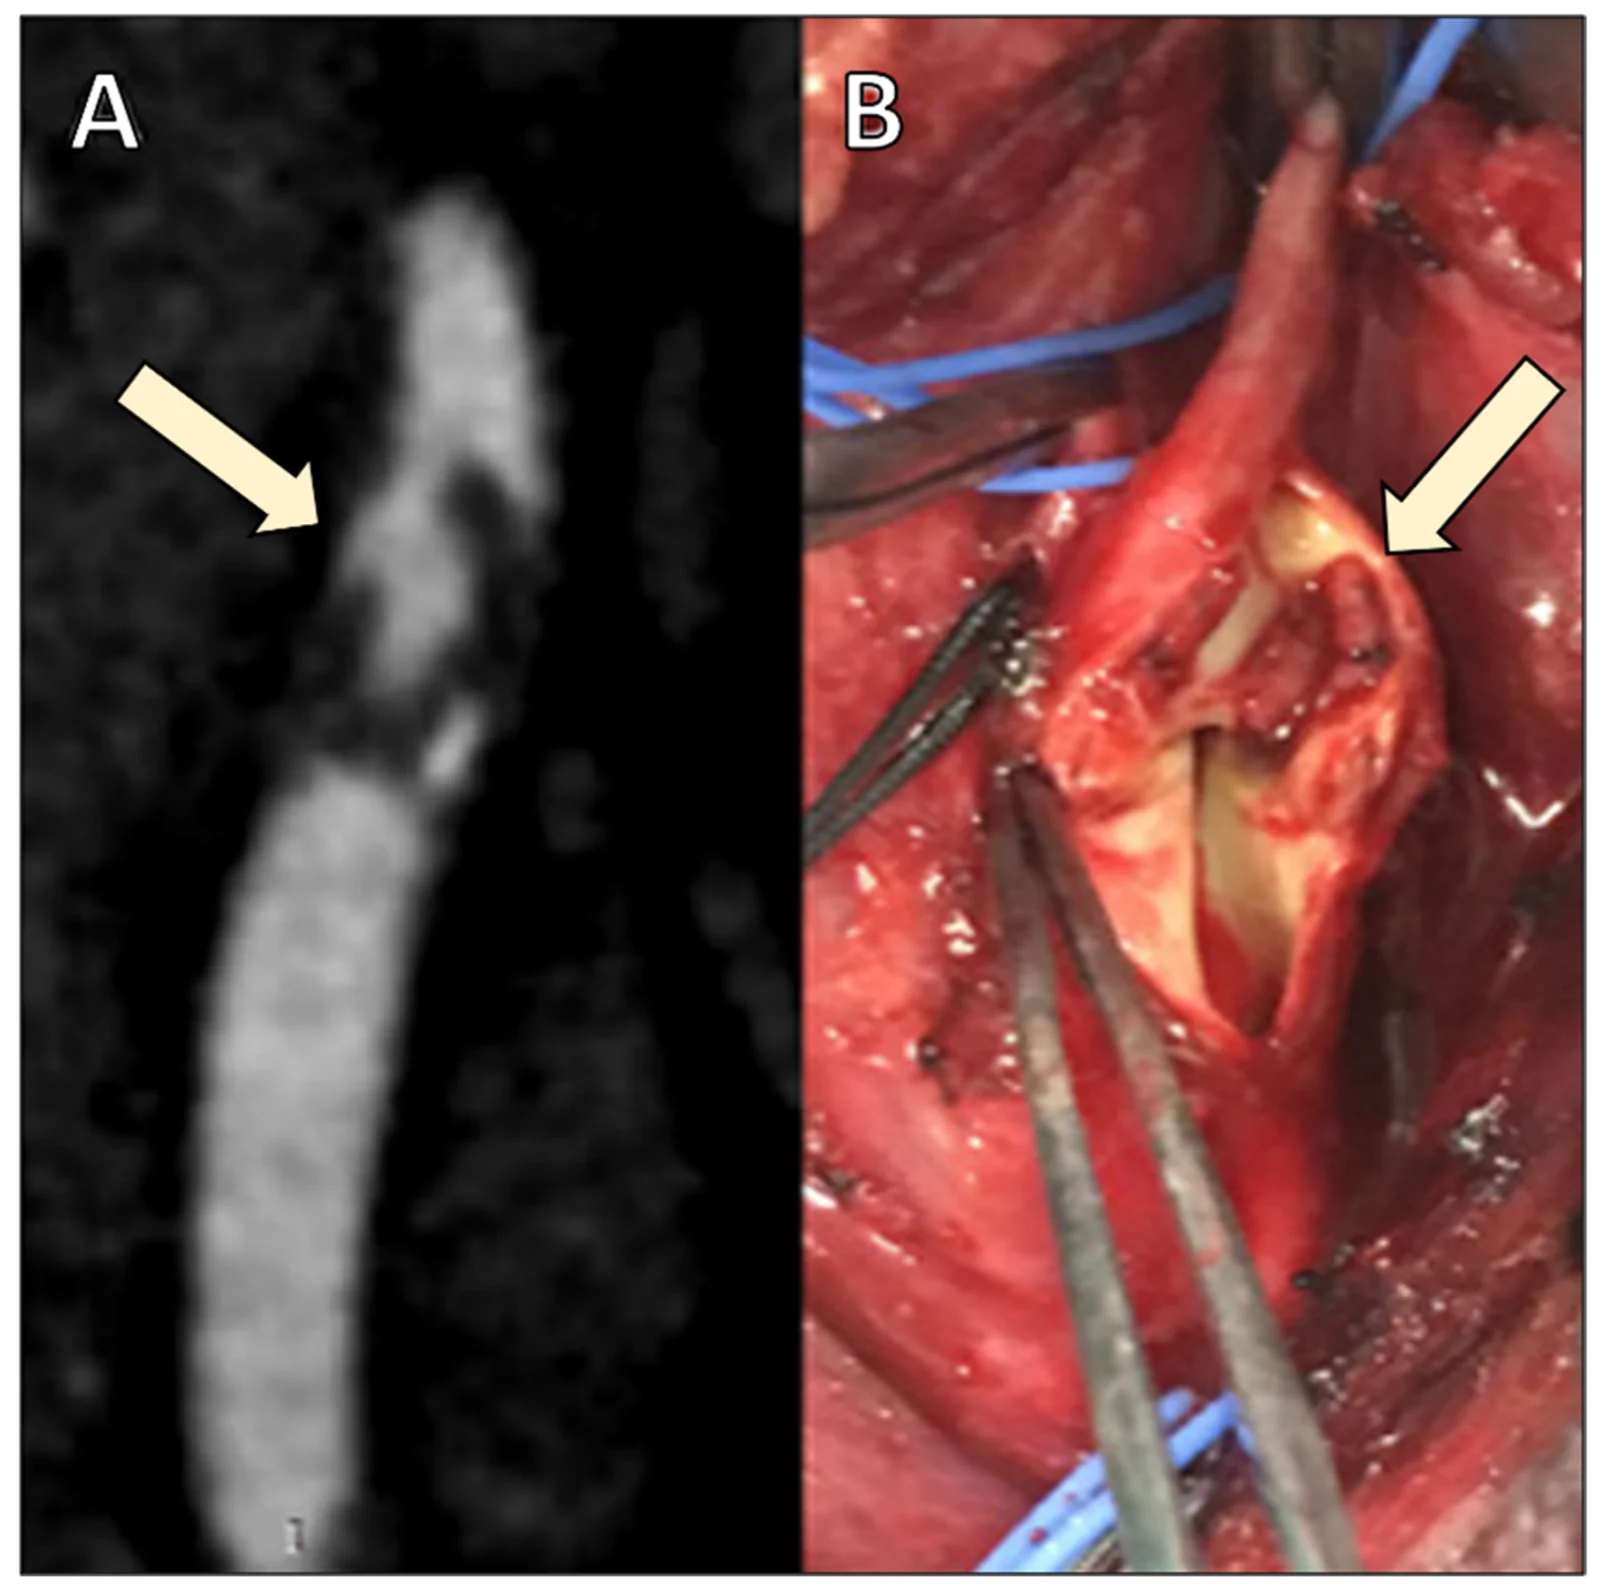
(A) Computed tomography angiography (CTA) demonstrating the characteristic “finger sign” of a carotid free-floating thrombus (CFFT) in the sagittal plane. (B) Intraoperative photograph obtained during carotid endarterectomy showing a free-floating thrombus arising from a small atherosclerotic plaque and extending into the lumen of the internal carotid artery.
